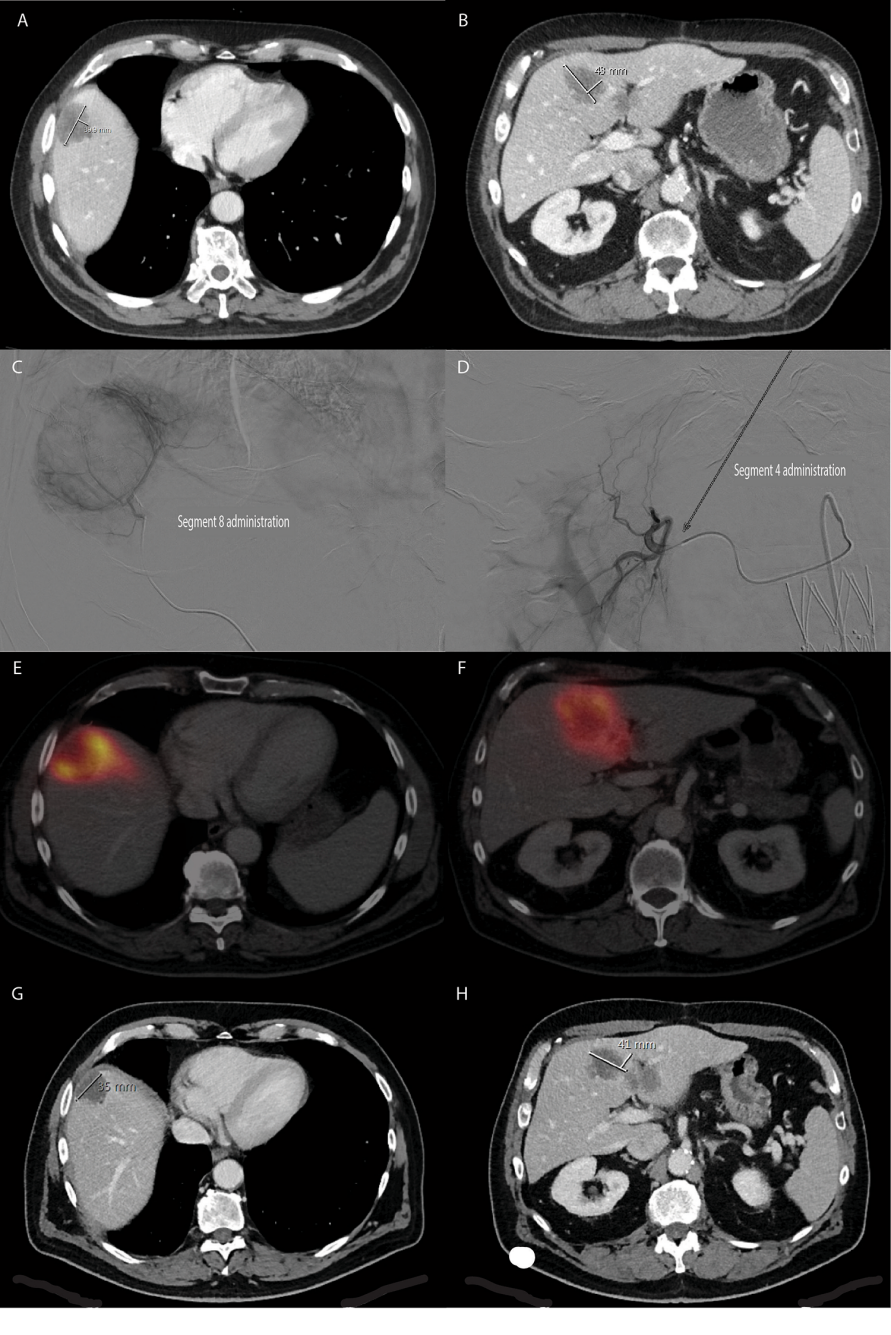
64 year old male diagnosed with intrahepatic metastatic cholangiocarcinoma (ICC), with distinct lesions in segment 8, 4 and a minor lesion on the edge of segment 3/4B (A and B). At tumor board, he was considered to be eligible for radioembolization treatment with ^166^Ho microspheres, which he received after an uneventful ^166^Ho scout procedure. On the day of treatment a superselective injection of 1.6 GBq (radiation segmentectomy) in segment 8 (C) and segmental injection of 0.8 GBq in segment 4 (D) was executed. Post-treatment SPECT/CT showed a good accumulation of particles around the tumor in segment 8 (E) and segment 4 (F). Contrast enhanced CT (G + H) and ^18^FDG-PET (not shown) acquired 2 months after treatment showed a near complete regression of the segment 8 lesion and partial response of the segment 4 lesion. Recent follow-up treatment (not shown) consisted of additional ^166^Ho radioembolization of segment 4 and superselective in segment 3. Segment 8 lesion is still in (near) complete remission
